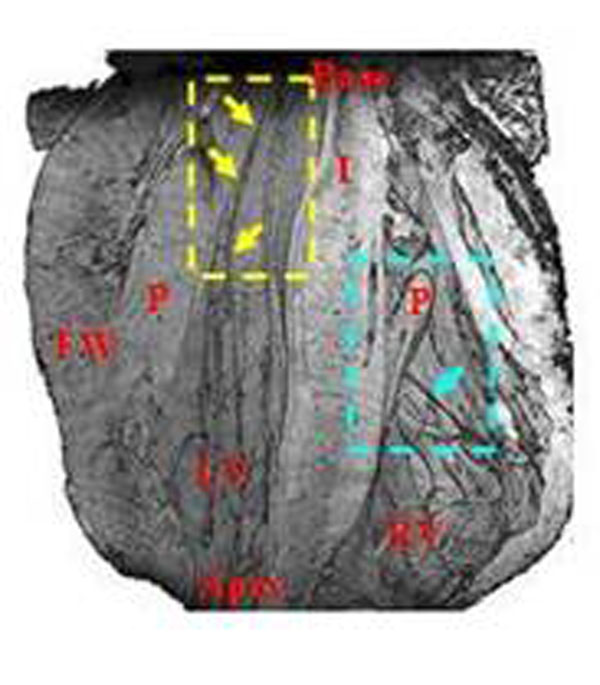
A representative volume rendered 3D MR image to visualize the conduction paths and anatomical features in an isolated heart. A red line and a green arrow in a red box indicate where sectioning occurred and viewer was located to perform the volume rendering. Note that free-running Purkinje fibers in the left ventricular cavities, a left bundle branch (yellow arrows) in ventricular inner wall, a right bundle branch (cyan arrow) and right bundle (right, yellow circle). LV: left ventricular cavity, RV: right ventricular cavity, leaflets of the mitral valve, FW: free wall, P: papillary muscle, I: ventricular interseptum.
